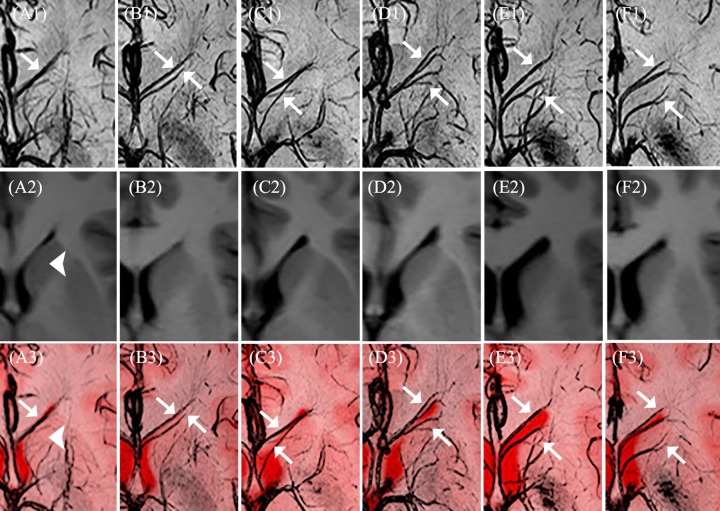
Different types of anterior septal veins. SW images (A1-F1) and the corresponding T1-weighted images (A2-F2) are fused to show the anterior septal veins and their drainage (A3-F3): A1-C1 and A3-C3 represent type 1, and D1-F1 and D3-F3 represent type 2. (arrow, anterior septal vein; arrowhead, caudate nucleus).
